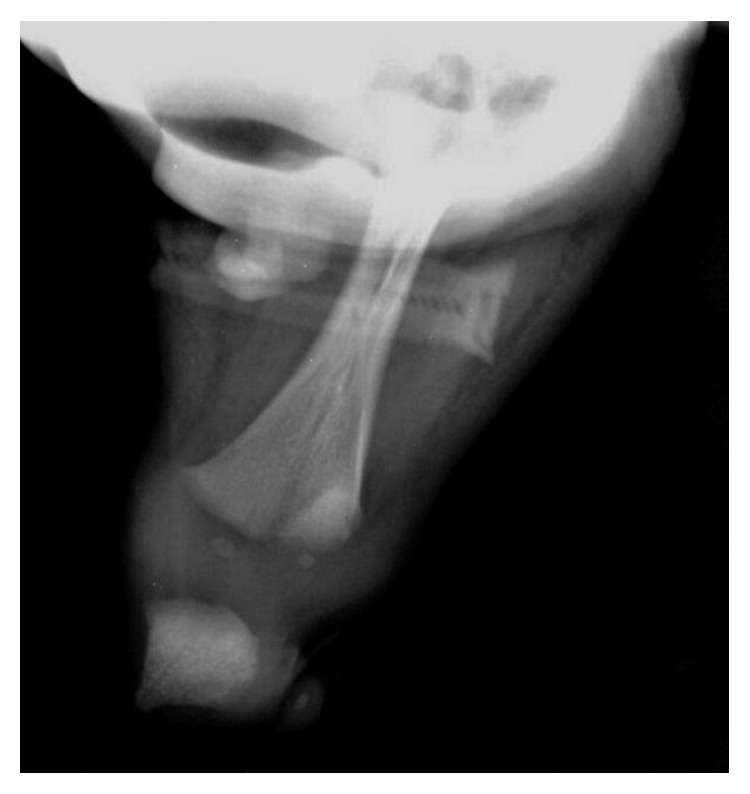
Lower limb X-ray showing complete fusion of both femurs.
